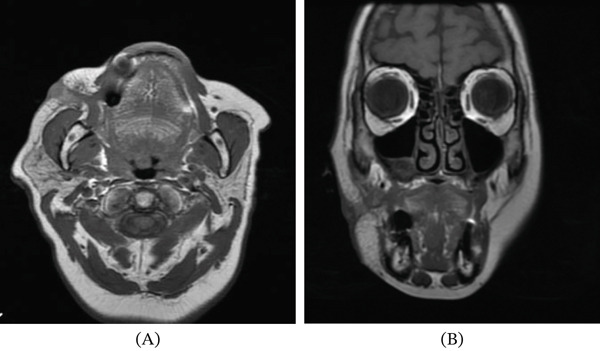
MRI images demonstrating a lesion originating from the right maxillary sinus adjacent to the lateral window sites. (A) Coronal view. (B) Axial view.
